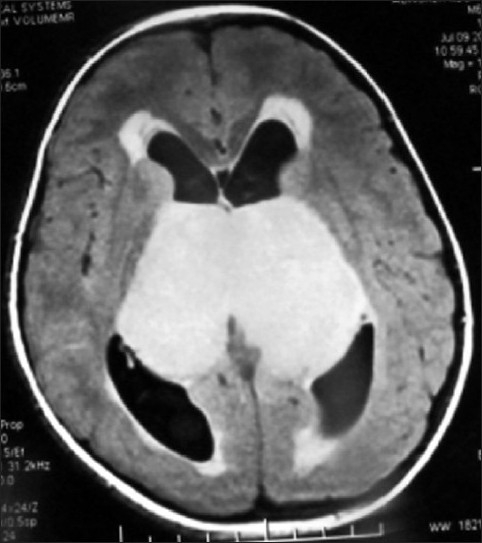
MR scan of head FLAIR image showing significant periventricular lucency with hydrocephalus
